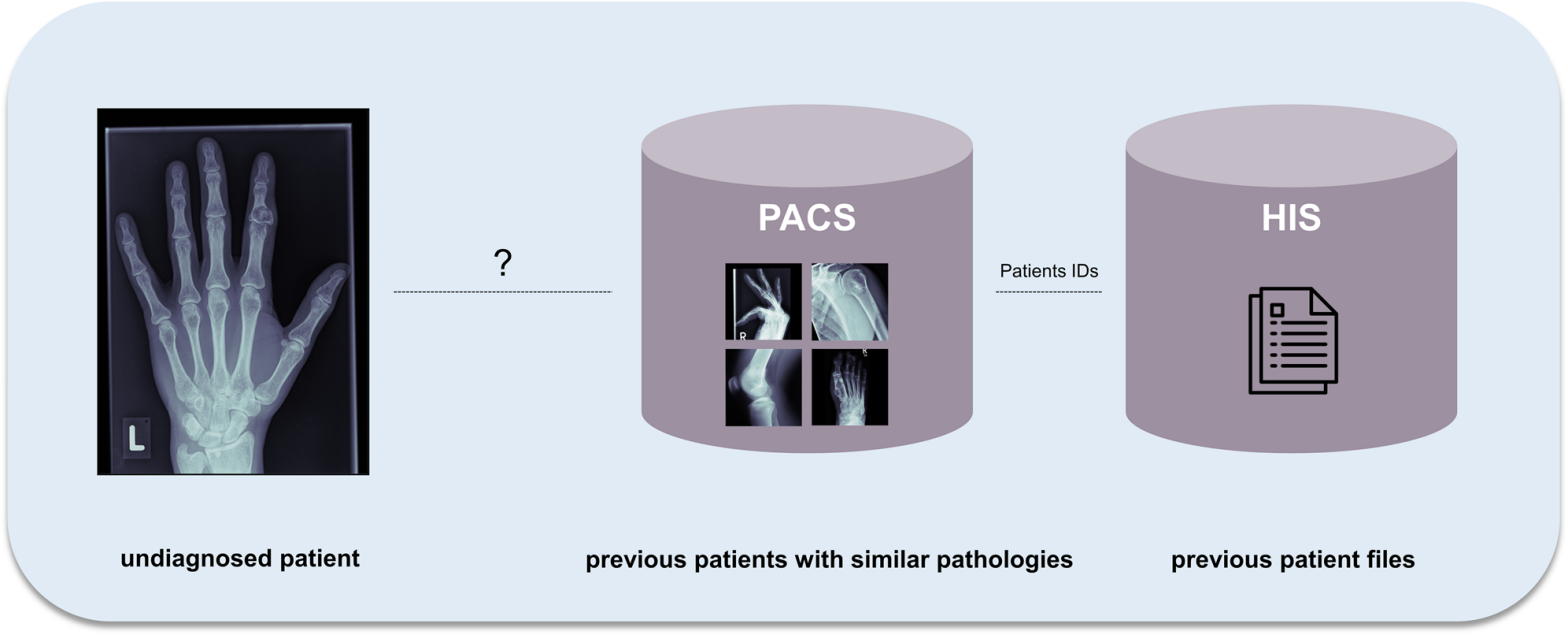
General concept of the proposed method—clustering new patients with previous patients based on radiographs to identify similar cases and classify tumour entity (PACS, picture archiving and communications systems; HIS, hospital information system)
